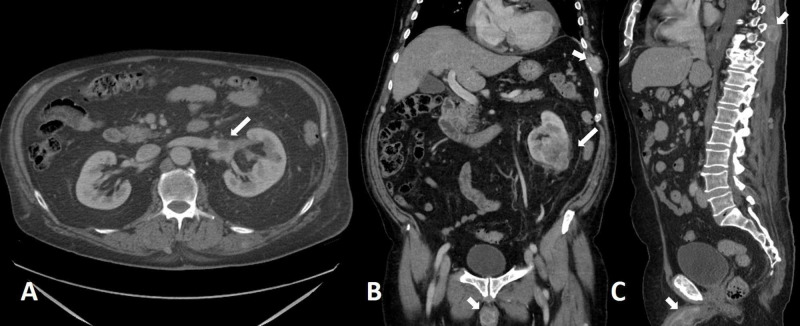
Clear cell renal cell carcinoma (long arrows) Computed tomography images taken in the axial (a), coronal (b), and sagittal (c) planes show a large heterogeneously enhancing left lower renal pole lesion with extension in the left renal vein (axial, long arrow). Sagittal and coronal reformats demonstrate penile and musculoskeletal metastatic disease (short arrows).
